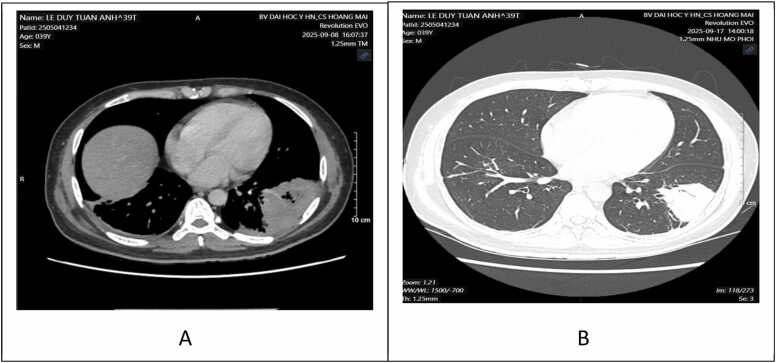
A) Chest CT scaner at the admission showed areas of consolidation at both lung with a large lesion containing a cavitary necrotic component on the left. B. Imaging on 11 days of treatment revealed a 44 × 41 mm mass in the left lower lobe, containing several fluid-filled bronchial branches, with heterogeneous enhancement after contrast administration (smaller lesion compared with image A).
